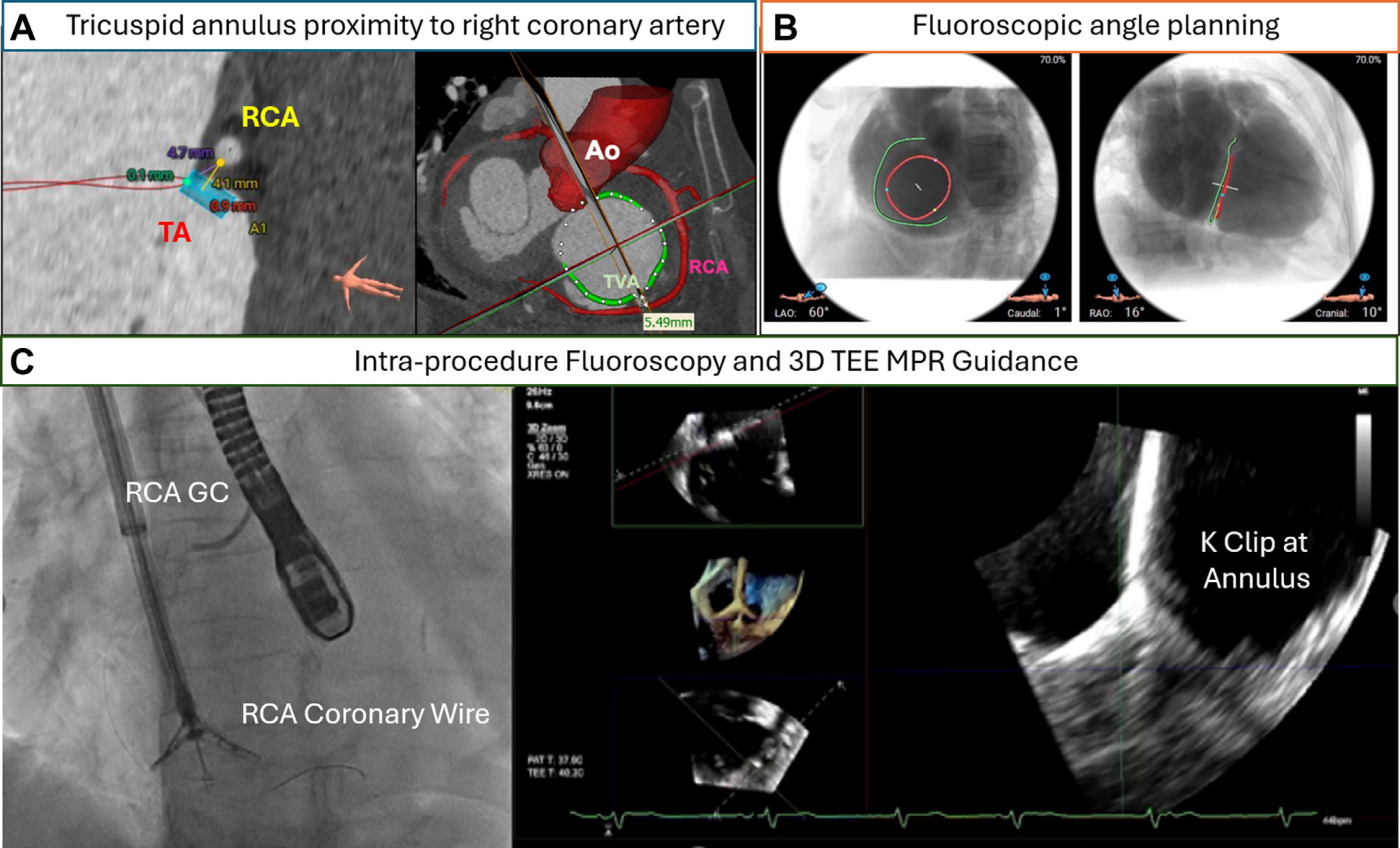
Multimodality Imaging to Guide K-Clip Transcatheter Annuloplasty (A) Preoperative computed tomography was performed to assess the tricuspid annulus (TA) dimension and the proximity of right coronary artery (RCA) to the TA. (B) Fluoroscopic projection for device implantation was determined. (C) During the procedure, an RCA guide catheter (GC) with a coronary wire is inserted to look for any coronary impingement by the K-Clip, and 3D TEE MPR is used to guide the steering and implantation of K-Clip. Abbreviations as in Figure 5.
